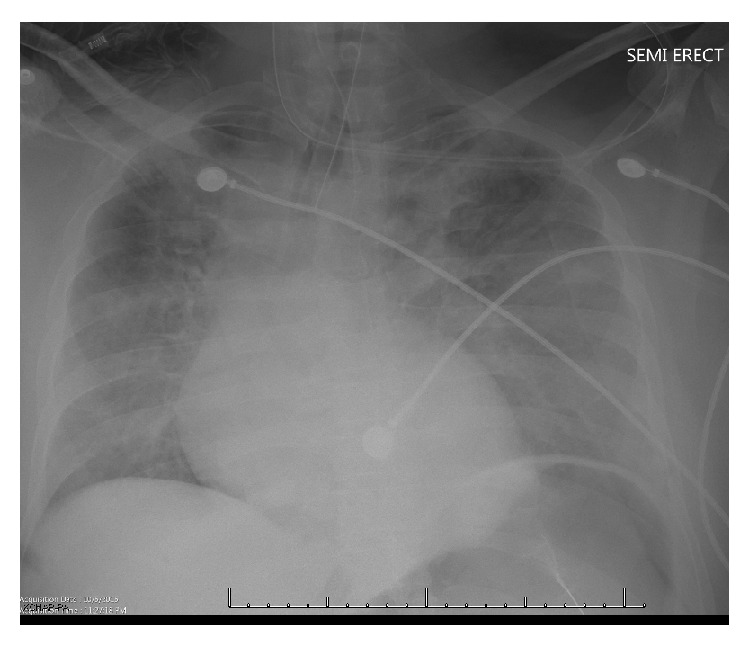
Chest radiograph shows endotracheal tube in position.
